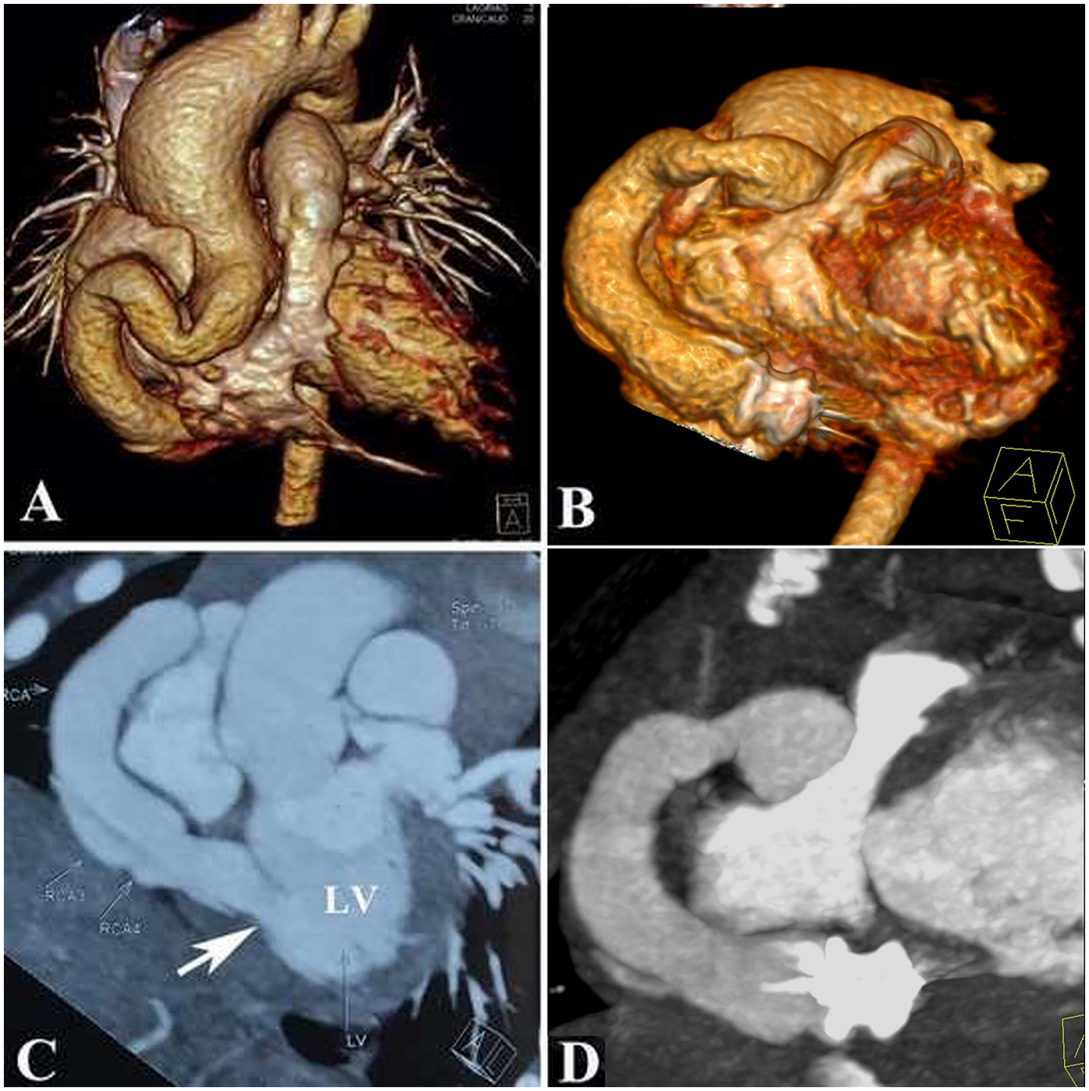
Anatomy of the coronary artery fistula showed by a computed tomographic angiography: (A,C): Pre-procedure; (B,D): Post-procedure.
